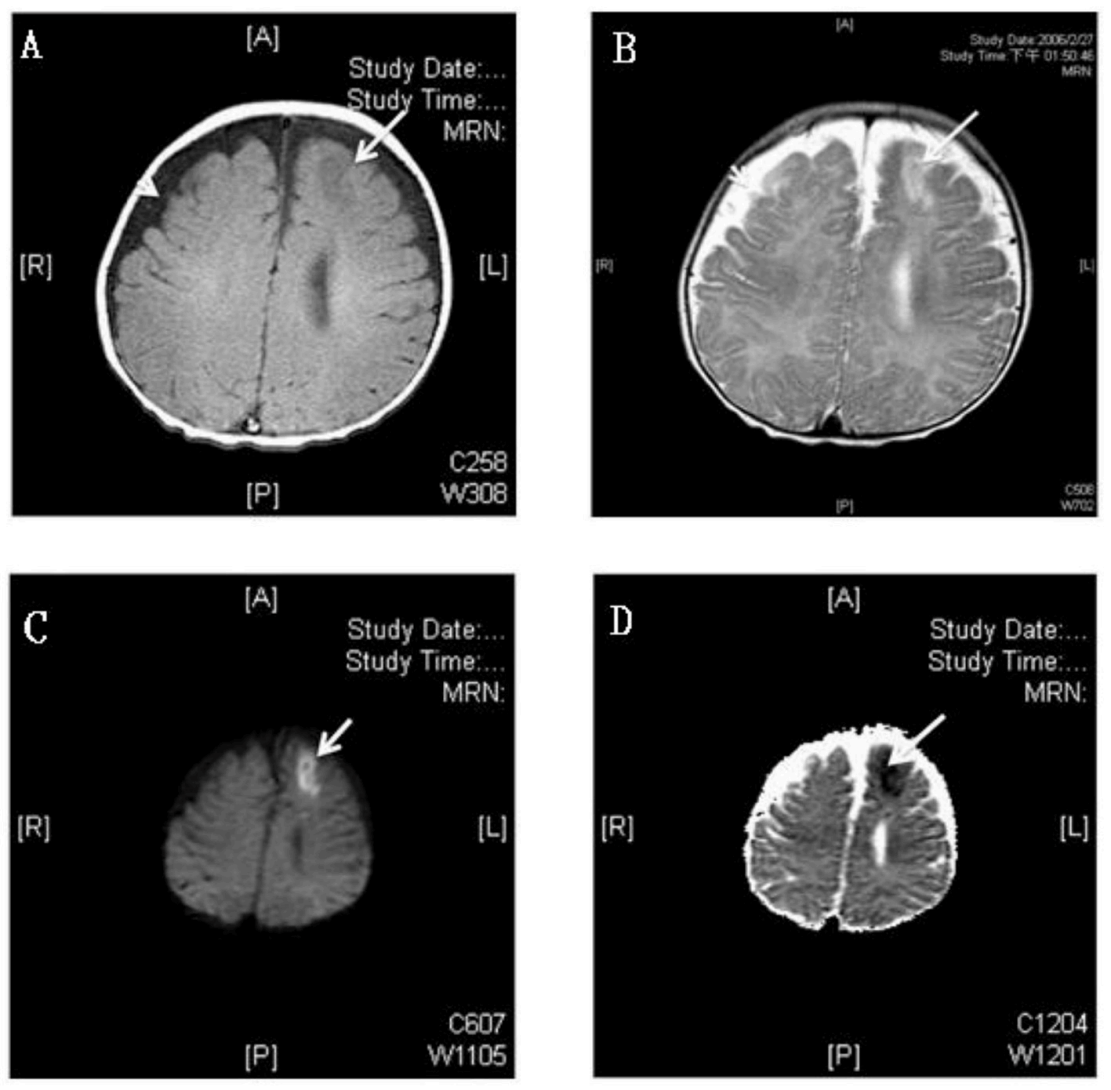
MRI study of a 3 month old boy with GBS meningitis and infarction. (A) Axial T1-weighted gadolinium-enhanced MRI showed hyperintensity and (B) T2-weighted MRI showed hypointensity over left anterior frontal area (white arrows) and bilateral frontotemporal subdural effusion (white arrow head); The left anterior frontal lesion showed hyperintensity over diffusion-weighted (C) and hypointensity over apparent diffusion coefficient (D) weighted MRI which revealed recent infarction (white arrows).
